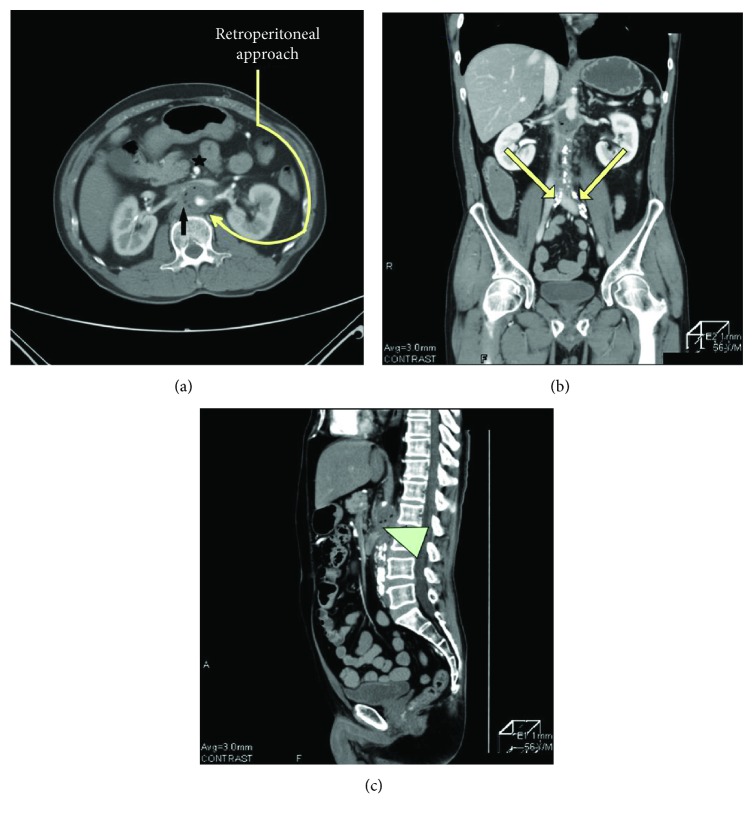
Preoperative enhanced computed tomography indicates an infected aortic aneurysm at the thoracoabdominal level. (a) Small aneurysm with intra-aortic air (arrow), the superior mesenteric artery (star), and renal artery in the axial view. (b) Obstructed distal abdominal aorta and common iliac arteries (arrows) with calcification in the coronal view. (c) Suspected aortoduodenal fistula (arrowhead) in the sagittal view.
